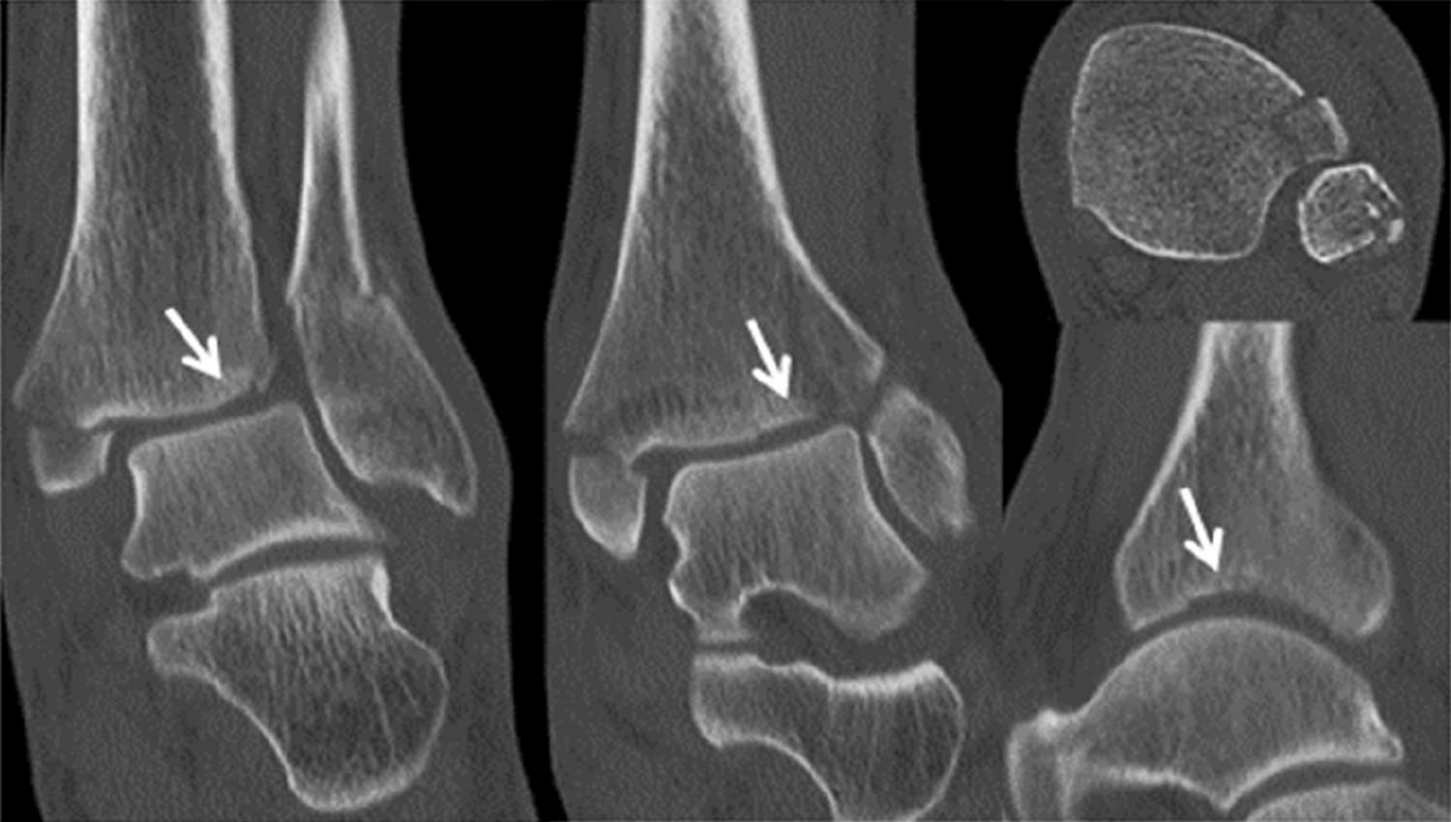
Preoperative CT imaging showing a type 3 anterior malleolar (Chaput) fracture with marginal impaction of the lateral plafond (arrow) as part of a trimalleolar fracture pattern with addition medial and lateral malleolar fracture (same patients in Fig. 1)
